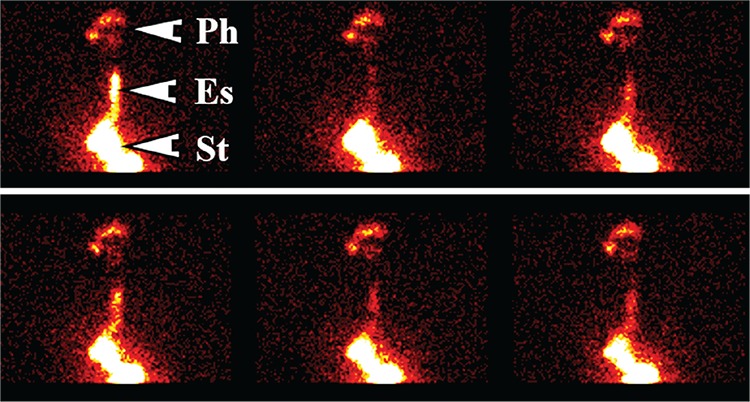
Dynamic sequence of the scintigraphic study showing full-column gastro-oesophageal reflux to the level of the pharynx. The oesophagus and stomach are labelled as Es and St, respectively
